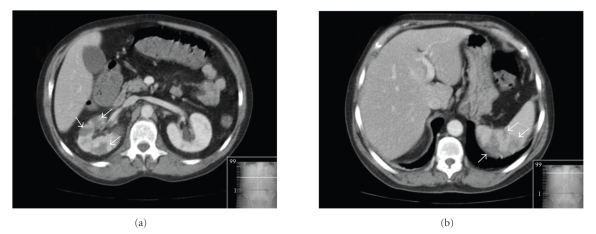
Abdominal computed tomography scan showing multiple ischemic areas (*arrows*) in the right kidney (a) and in the spleen (b), suggesting an embolic source.
